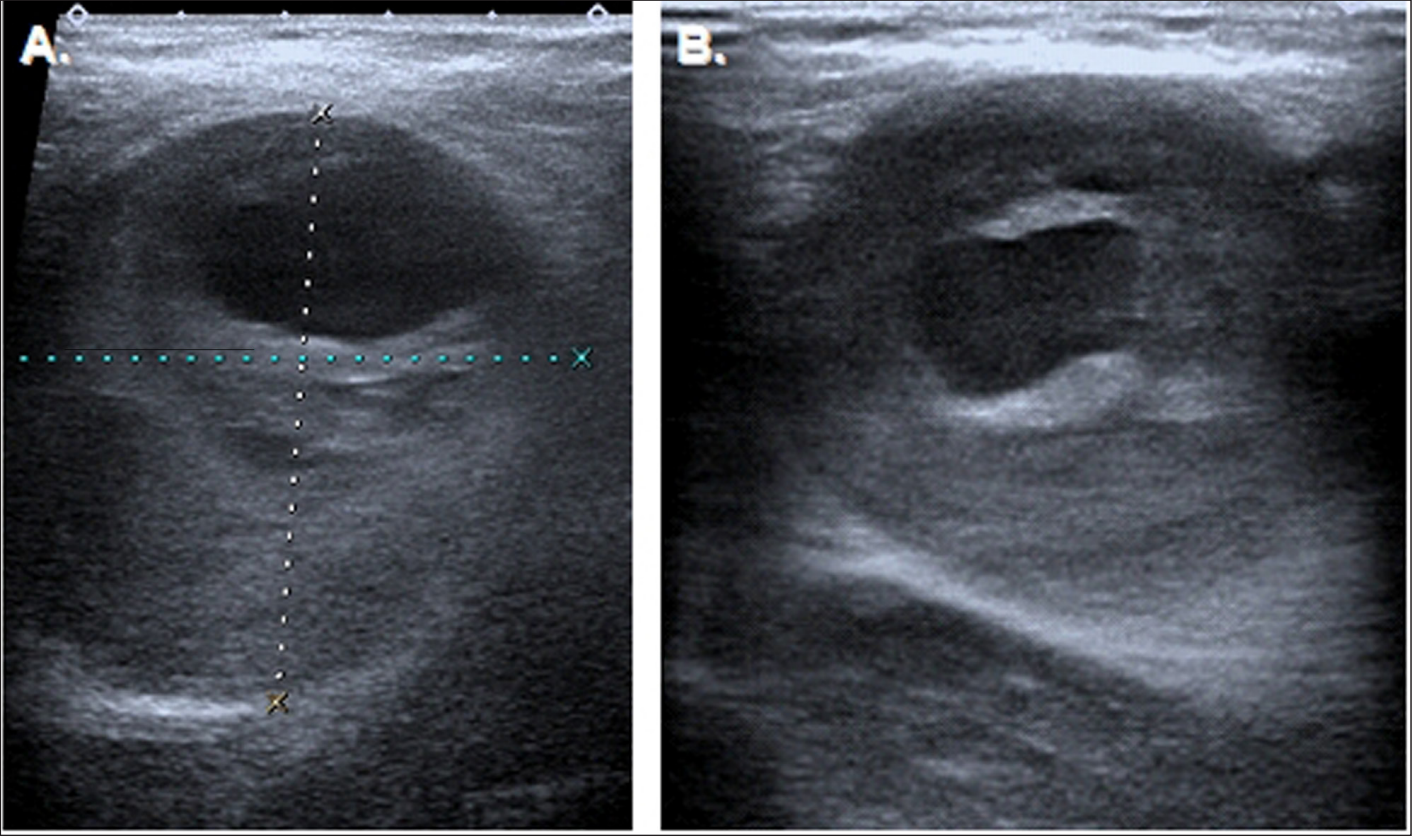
Ultrasonography of the axillary masses. (A) Transverse section show 67 × 45 mm partially thrombosed aneurysm on the right, (B) 40 × 45 mm partially thrombosed aneurysm on the left.
